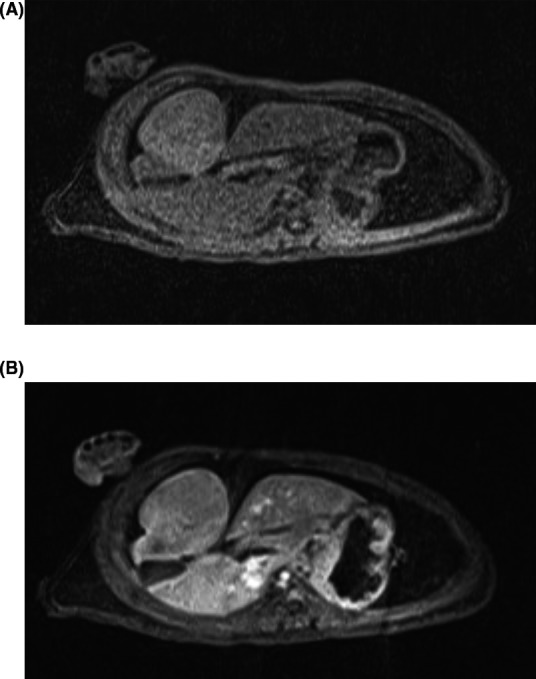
MRI findings. A, T1‐weighted MRI image of a woodchuck HCC demonstrates an exophytic right lobe mass. B, Post‐contrast images demonstrate the lesion to be isoenhancing versus background liver
